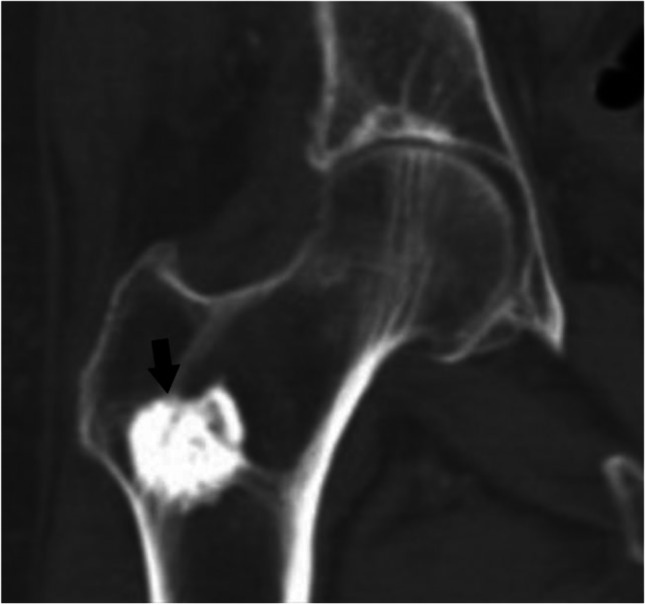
Case 2. Enostosis. 68-year-old female presents with right pelvic pain after a fall. Coronal CT image shows a densely sclerotic lesion (arrow) with spiculated margins in the proximal femur with mean density = 1644 HU
